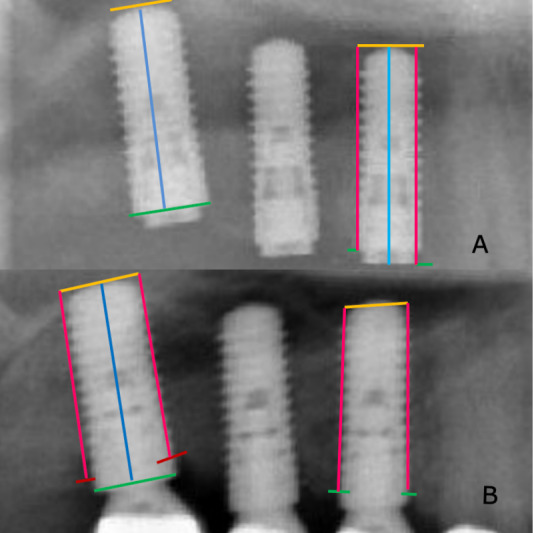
A: Postoperative radiograph after insertion of 3 implants in the posterior maxilla region with representation of the measurement procedure for determining the initial findings. B: Radiograph of the same area at the 5-year follow-up examination with representation of the measurement procedure for determining peri-implant bone resorption. Green line: initial bone height; blue arrow: implant length; orange line: apical implant edge; pink arrow: measured distance from the crestal bone to apical implant edge; red line: crestal bone line after peri-implant bone resorption
